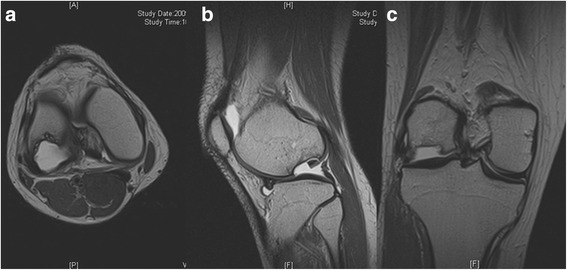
Preoperative magnetic resonance image. a Axial, b sagittal and c coronal images showed large osteochondral defect (approximately 2.7 cm × 2.2 cm sized and 1.5 cm deep) on lateral femoral condyle with osteochondral loose body
